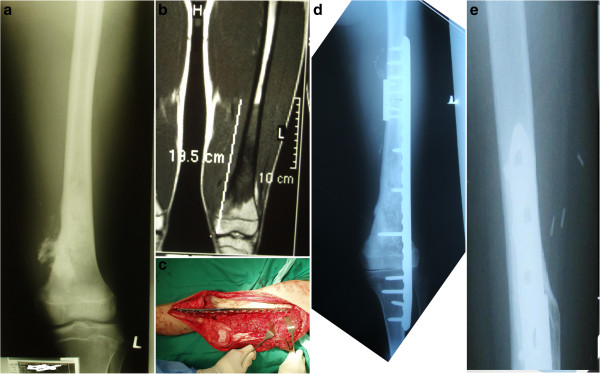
Patient 2: 14-year-old boy with osteosarcoma of the distal femur. (A) Plain radiograph showing changes at the distal femur. (B) Magnetic resonance imaging scan showing the intramedullary extent of the tumor (180 mm). A further 30 mm was resected proximally during surgery, making a total of 210 mm of bone that was autoclaved and replaced. (C) Intra-operative photograph showing the autoclaved tumor bone placed in its original bed. (D) Post-operative radiograph showing fixation of the autoclaved tumor bone with a long locking plate and a 3.5 dynamic compression plate at the proximal junction. (E) Follow-up 14 months later; bone union and full weight-bearing without aids.
